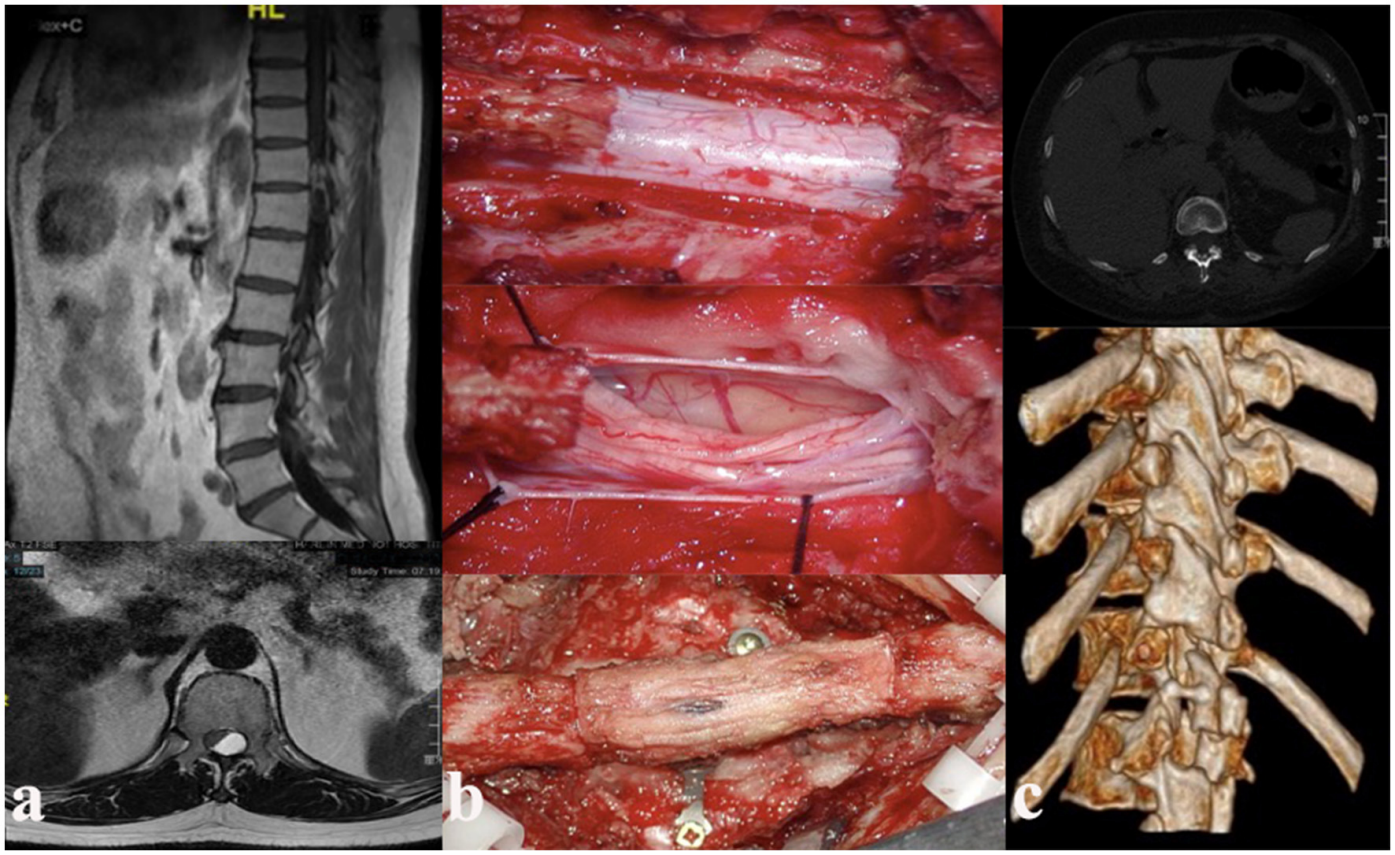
(a) Preoperative thoracic magnetic resonance imaging T1-weighted sagittal (up) and axial (down) image with contrast showing heterogeneously enhancing intradural extramedullary lesion at the level of the T11–12 vertebrae. (b) Intraoperative laminoplasty with resection of the laminae (up), exposure and resection of tumor (middle), and reduction of the resected lamina (down). (c) Postoperative computed tomography (CT) scan study.
